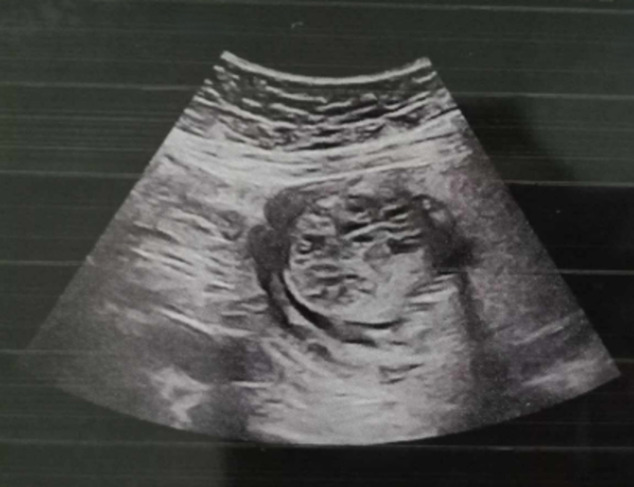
a prenatal ultrasound showing symmetrical bilateral nephromegaly with poly-cystic renal dysplasia
